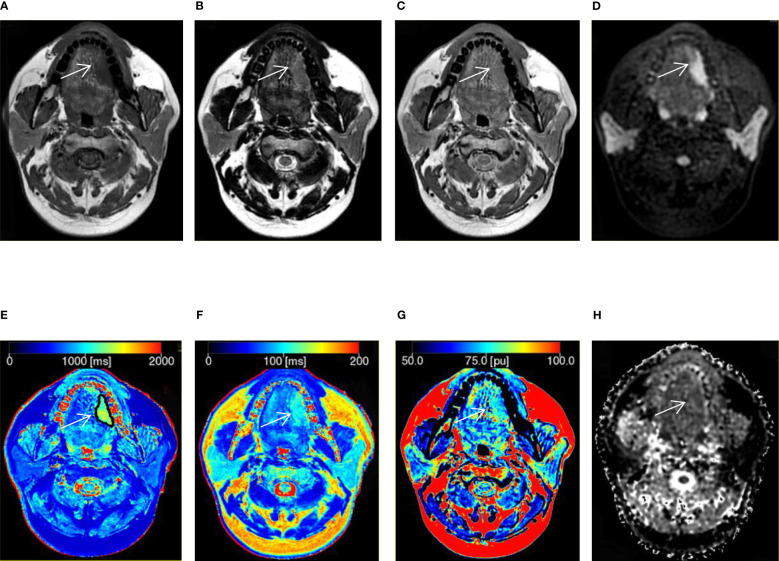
A 41-year-old man with squamous cell carcinoma in the tongue. (A–C) T1, T2, and proton density (PD) weighted images obtained from synthetic MRI. (D) Stimulus and fast spin echo diffusion-weighted imaging with periodically rotated overlapping parallel lines with enhanced reconstruction (b = 800 s/mm^2^). (E–G) Synthetic MRI-derived maps indicate that the mean T1, T2, and PD values measured by the two radiologists were 1214.67 ms, 105.17 ms, and 80.39 pu, respectively. (H) ADC map indicates that the mean apparent diffusion coefficient (ADC) value measured by the two radiologists was 1.52 × 10^-3^ mm^2^/s.
